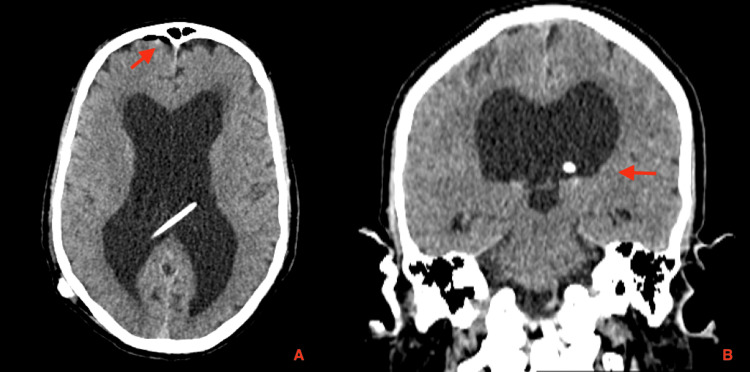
Postoperative head CT scan demonstrating appropriate placement of the ventricular catheter within the right lateral ventricle. (A) Axial CT image showing the ventricular catheter tip in optimal position, with a small amount of right frontal subdural pneumocephalus likely related to the ventricular puncture (red arrow). (B) Coronal CT image confirming correct intraventricular positioning. CT: computed tomography
